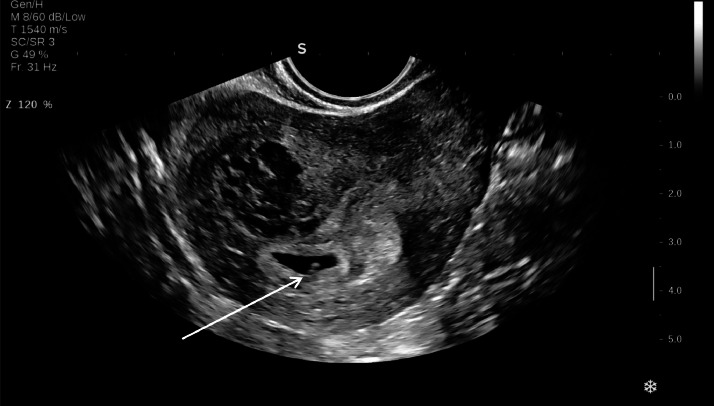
Transvaginal ultrasound showed an intrauterine pregnancy, containing a FP with FHR (white arrow).
